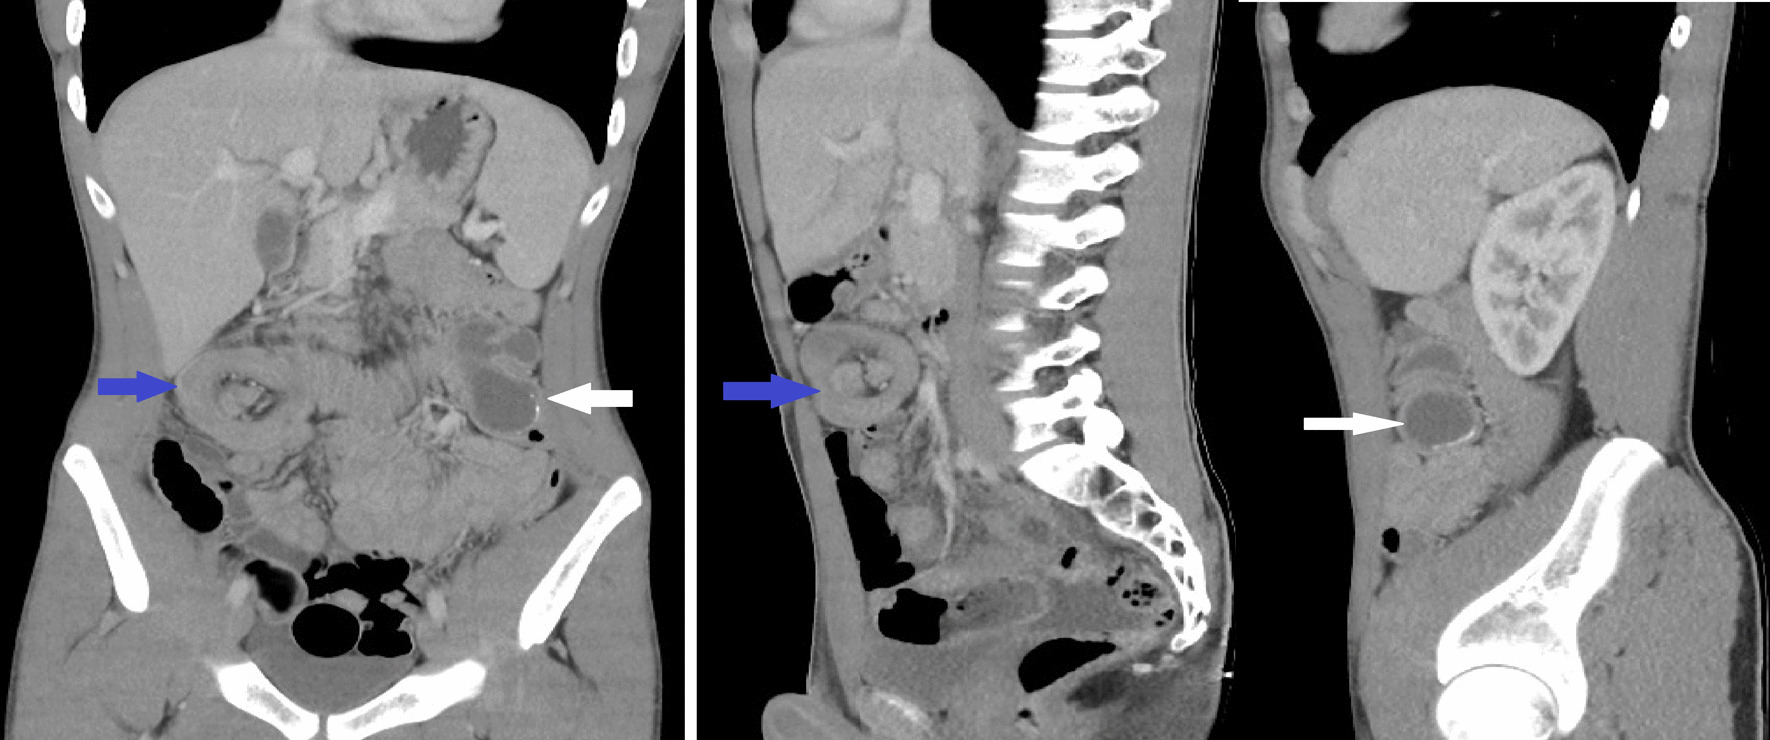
Frontal and longitudinal CT scan demonstrates the target or sausage-shaped lesions (blue arrows) and large appendiceal mucocele (white arrows)
